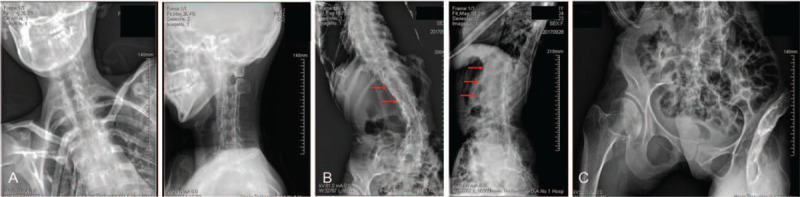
Radiographs of spine and sacroiliac joints. (A) Loss of physiological curvature of the cercal spine. (B) Kyphoscoliosis of thoracic and lumbar spines with ossification of the anterior longitudinal ligament. (C) Fusion of bilateral sacroiliac joints.
